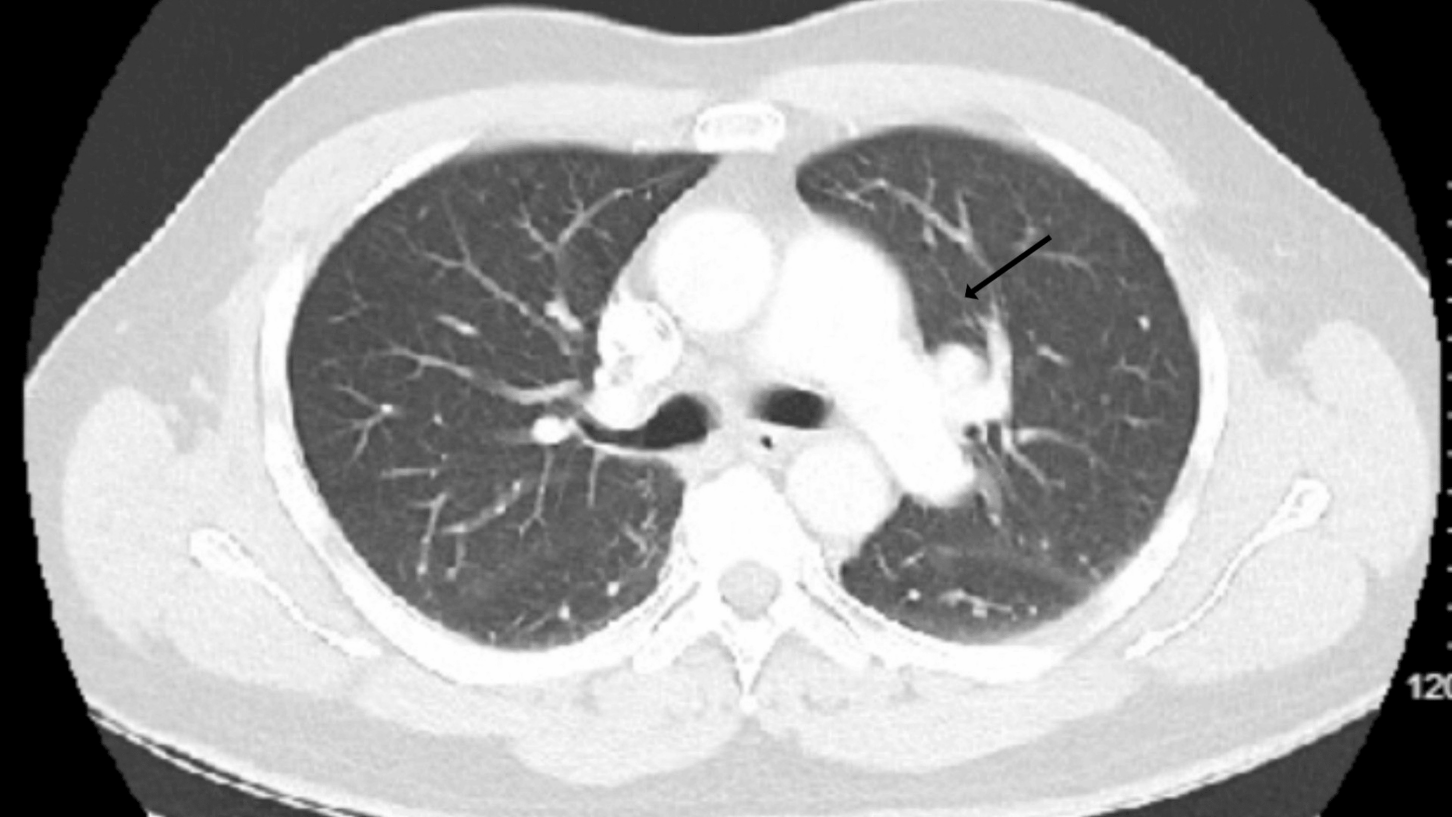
Chest CT showing resolution of the lesion after treatment
